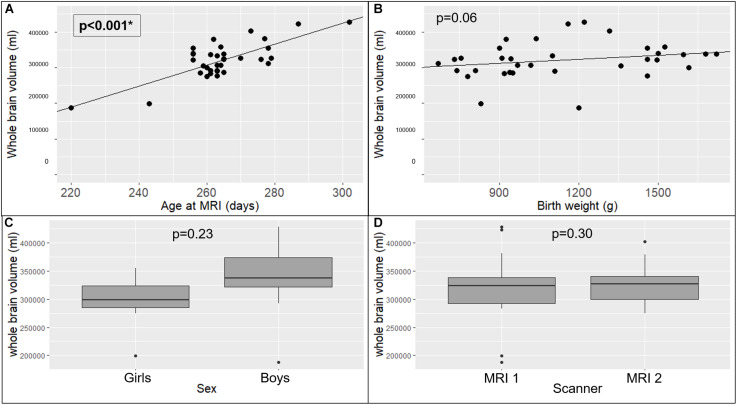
Effects of explanatory variables included in the multiple linear regression model on whole brain volume: age at MRI (A), birth weight (B), sex (C), and MRI scanner used (D). The significant *p*-values are in bold characters within a square frame.
